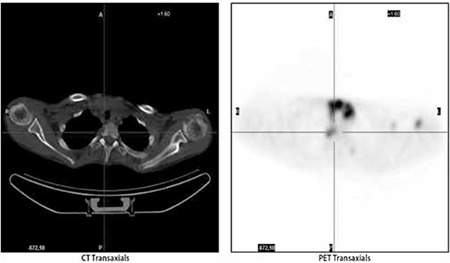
Axial CT part of the PET/CT study showing soft tissue involvement adjacent to the T2 vertebra
